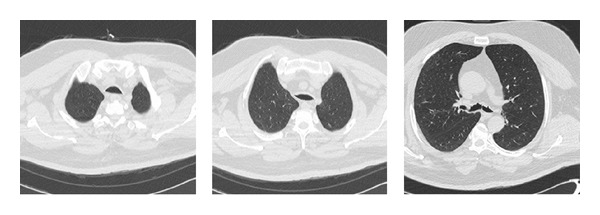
Thoracic computed tomography: in the airway segment starting from the proximal end of the trachea and extending to the main bronchi, an increase in the transverse diameter, a decrease in the anterior-posterior diameter, and diverticular irregularities in the level of the carina are observed.
